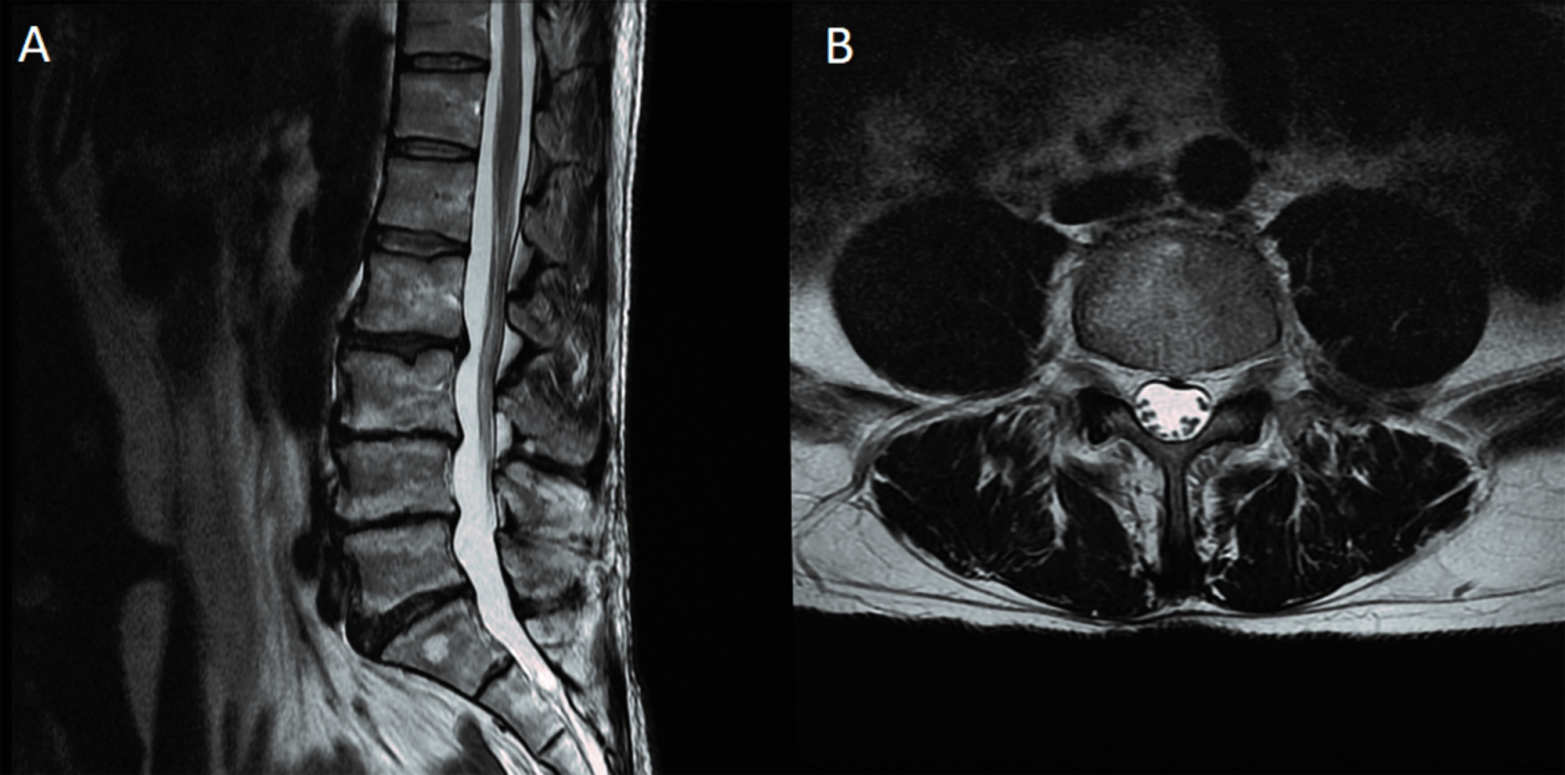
T2 MRI. A) Sagittal cut clearly showing no significant central stenosis; B) Axial cut at the L4-L5 level demonstrating no evidence of neuroforaminal stenosis at this level MRI: magnetic resonance imaging
